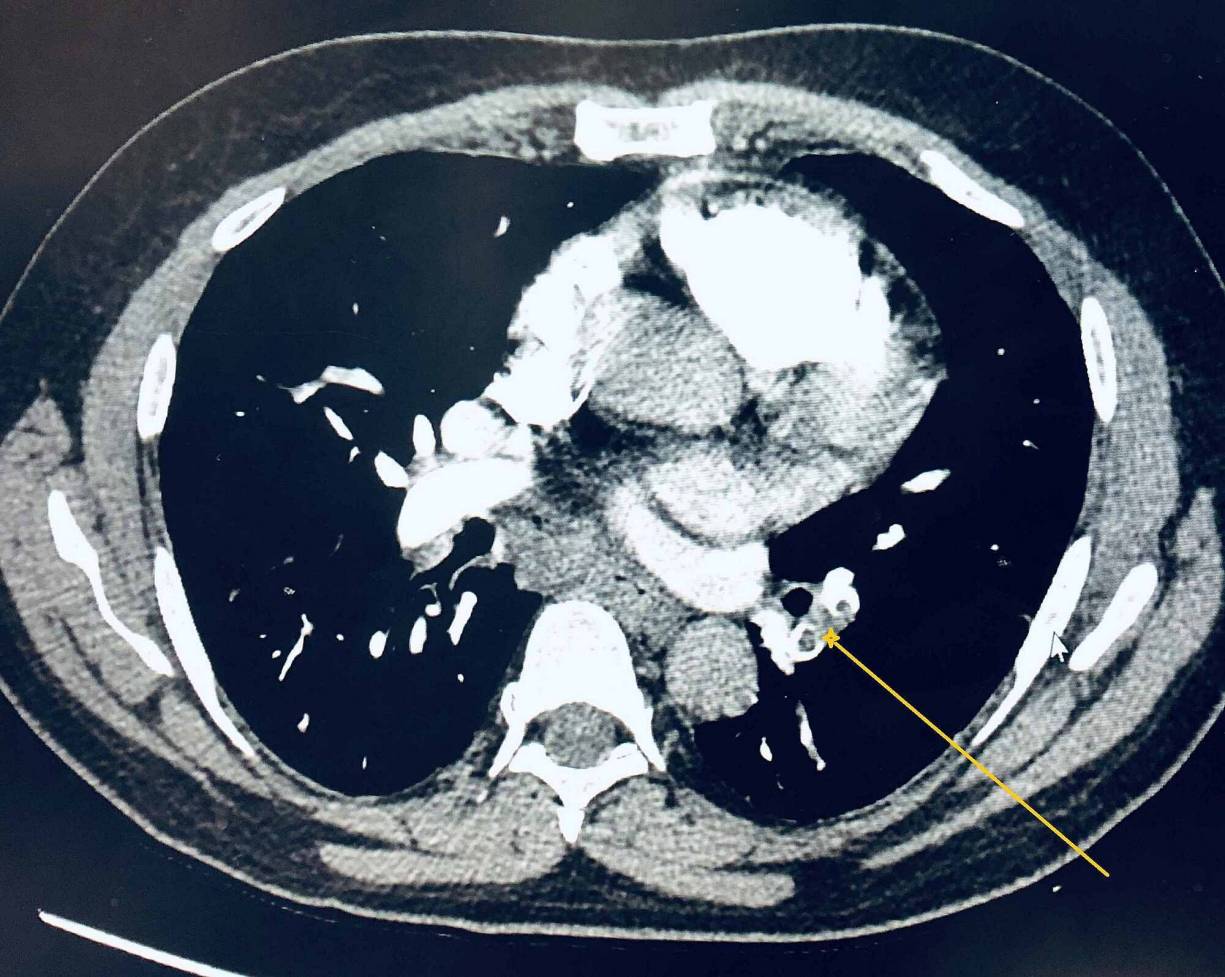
CTA chest axial view revealing acute appearing pulmonary emboli (pointed by yellow arrow) identified throughout multiple segmental branches of the left lower lobe pulmonary artery CTA: computed tomography angiography
